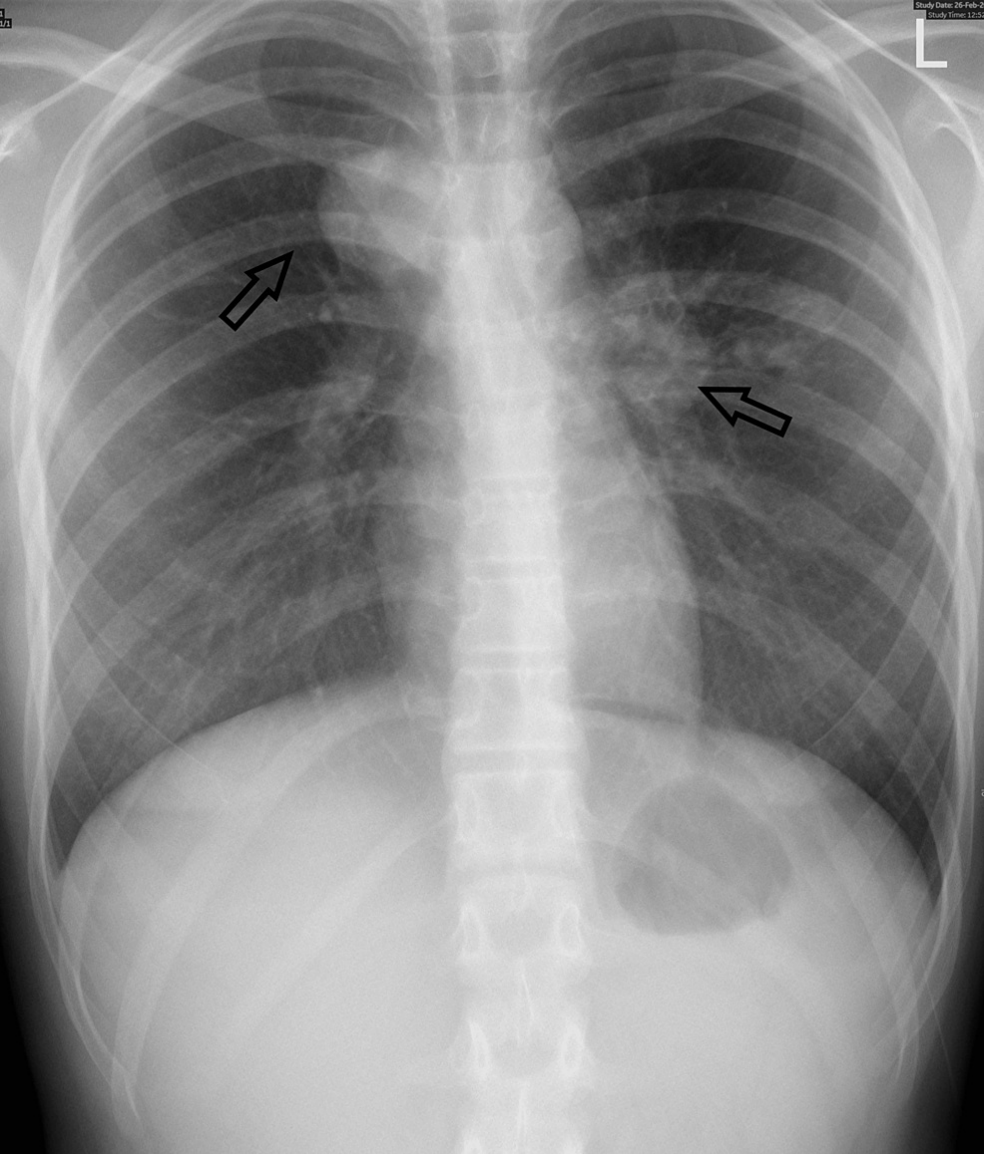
Repeated chest x-ray showed an increase in the volume of the hilar adenopathy and enlargement of the mediastinum (black arrows).
